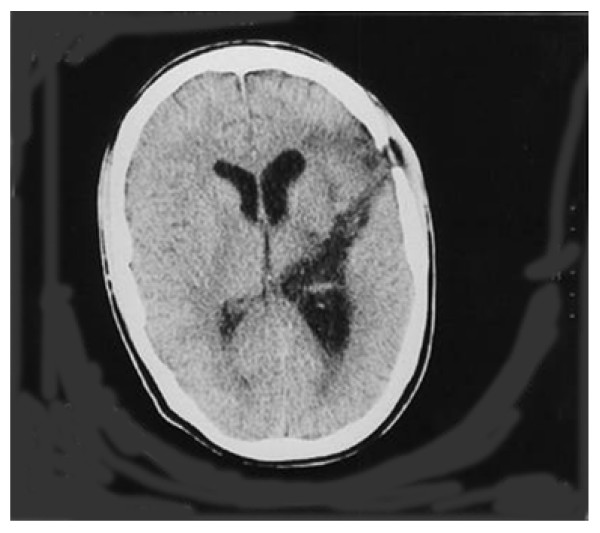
Axial CT scan of a 21 year old male who was stabbed in the left temporal lobe. Scan was taken 4 weeks post-injury and showed no difference from original scans (adapted from Connolly et al., 1999).
